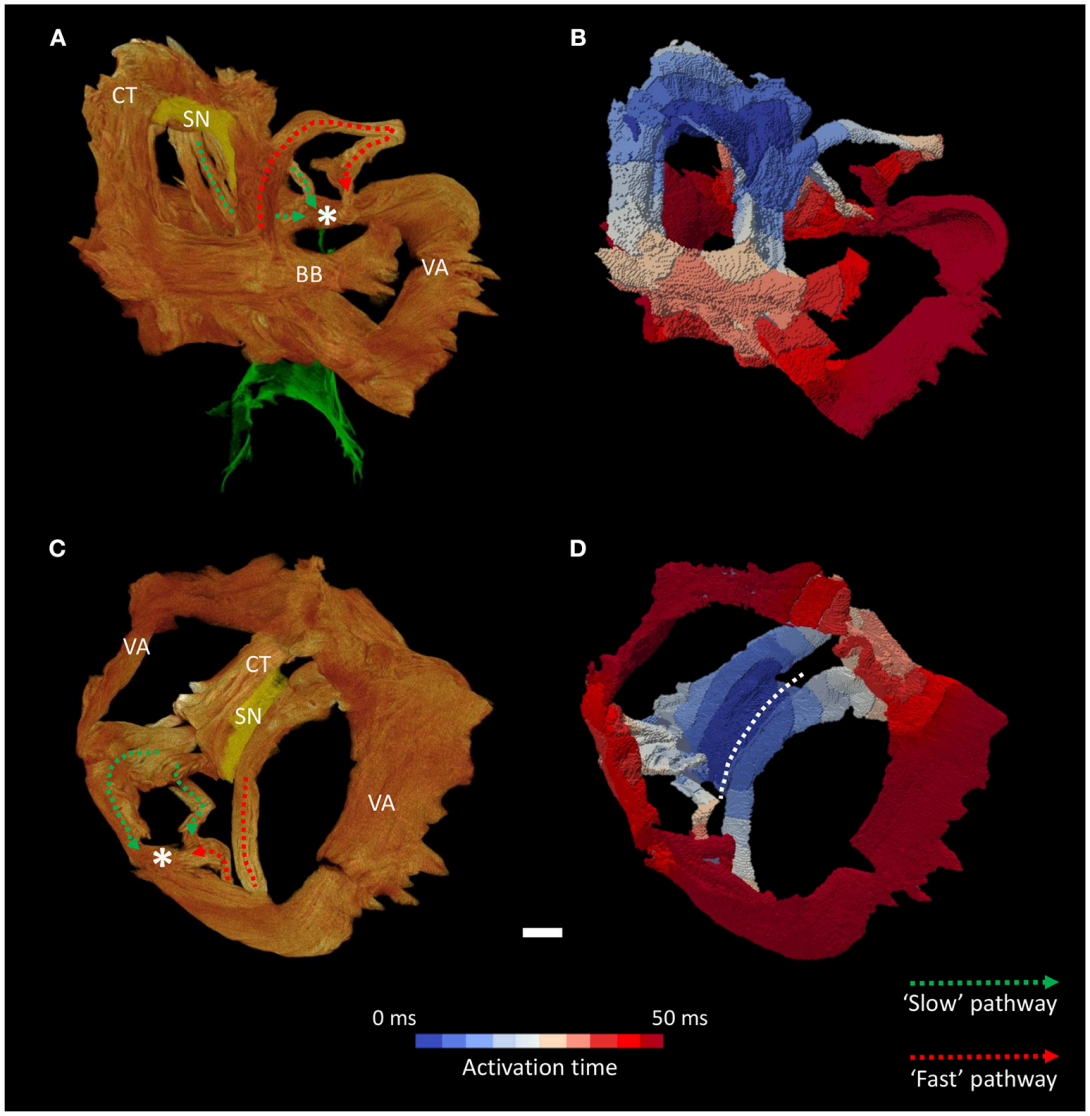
Inter-nodal conduction through the atrial muscle bundles II. Volume renderings of the atrial muscle bundles in anterior (A) and inferior (C) views, the normal location and direction of the “slow” pathway (green), and the septal aspect of the elongated “fast” pathway (red) are indicated by dotted arrows. (B,D) Display the corresponding electrical activation maps, showing excitation of the distal aspect of the region normally associated with the “slow” pathway precedes that of the “fast” pathway. See methods for modeling parameters. *Location of compact atrioventricular node; BB, Bachmann's bundle; CT, terminal crest; SN, sinus node; VA, valve annulus. Scale bar represents 3 mm.
